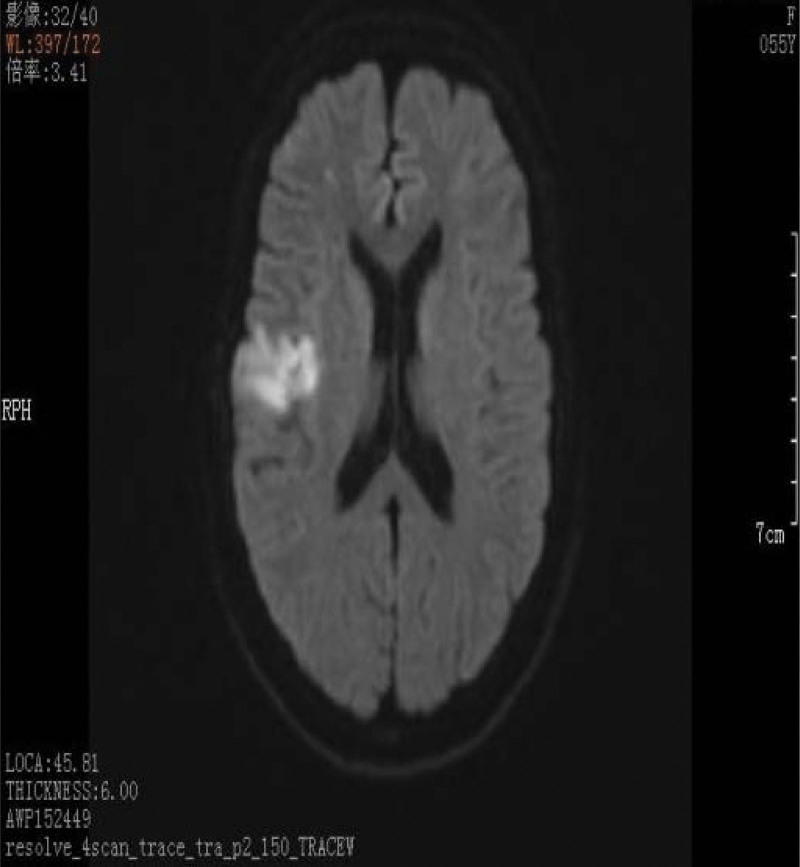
Diffusion-weighted magnetic resonance imaging of the brain showing acute cerebral infarction in the area concerning the right temporal parietal lobe.
